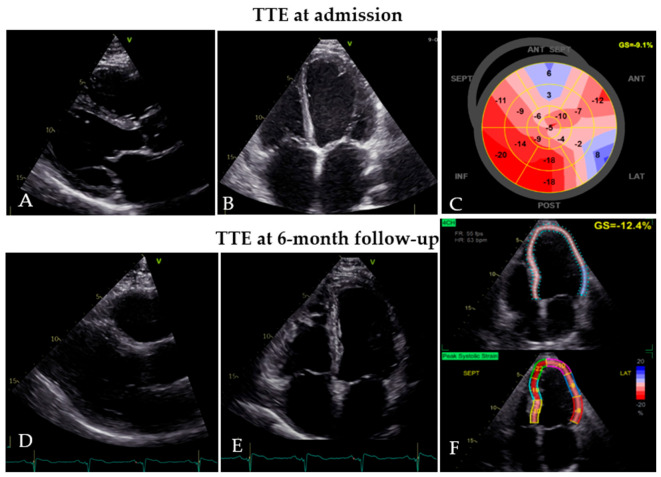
TTE at baseline and 6-month follow-up. (A) Parasternal view with LV dimension measurement; (B) apical 4-chamber view; (C) baseline global longitudinal strain bull’s-eye; (D) parasternal view at 6 months with slightly improved LV dimension; (E) apical 4-chamber view; (F) follow-up strain evaluation showing a modest recovery.
